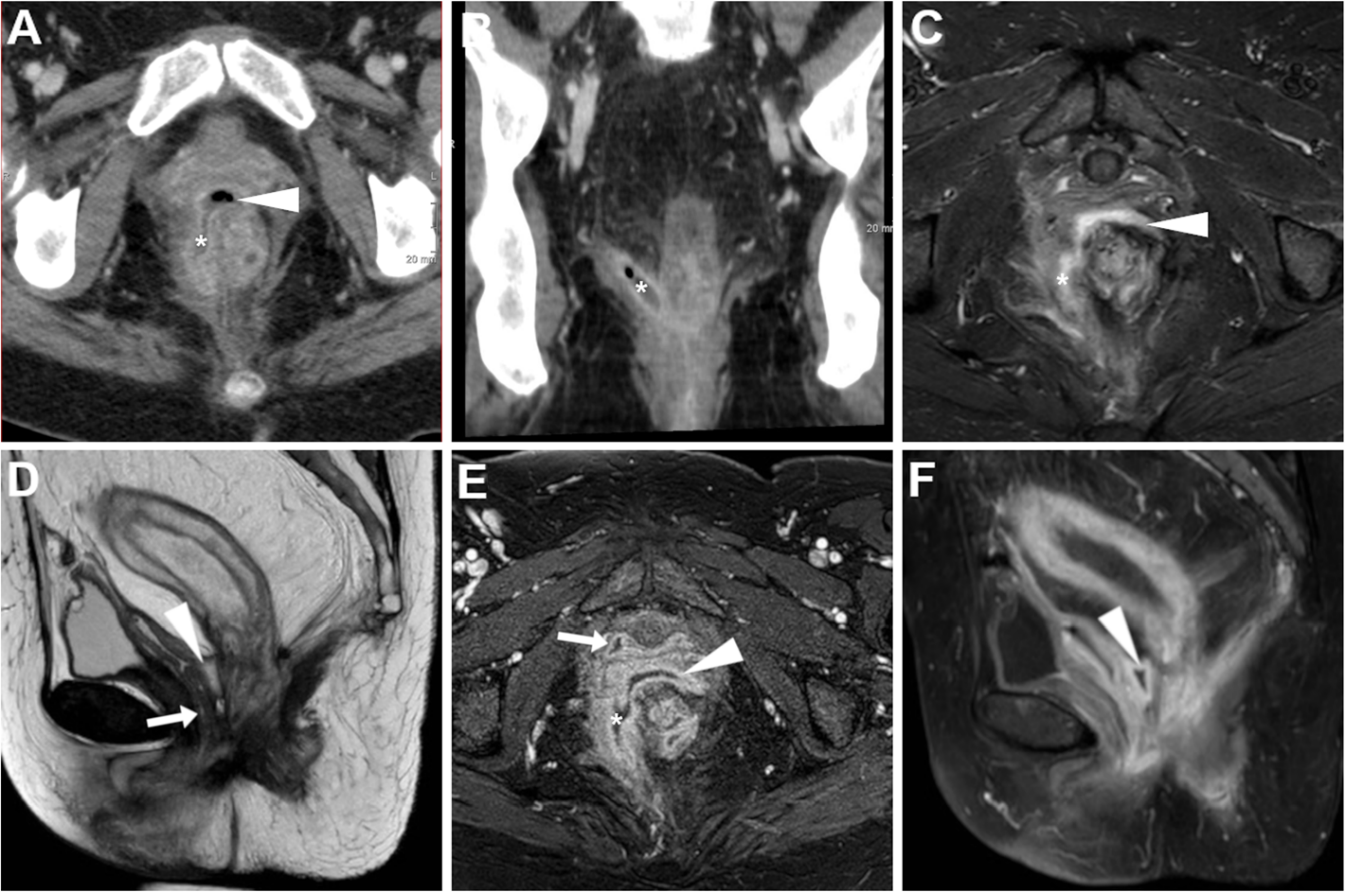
MRI-like use of CT to investigate CD-related perianal inflammatory disease. In a 50-year-old, small-FOV axial (a) and oblique-coronal (b) CT reformatted images show peripherally enhancing abscess collection extending from the anovaginal septum (arrowheads) to the right levator ani muscle (*). Axial FS (c) and sagittal (d) T2-weighted, post-gadolinium FS axial (e) and sagittal (f) T1-weighted MRI images show same findings with fluid-filled abscess cavities with strong mural enhancement. Note severe proctitis as circumferential thickening of rectal walls with oedematous submucosa (in d) and marked homogeneous enhancement (in f)
